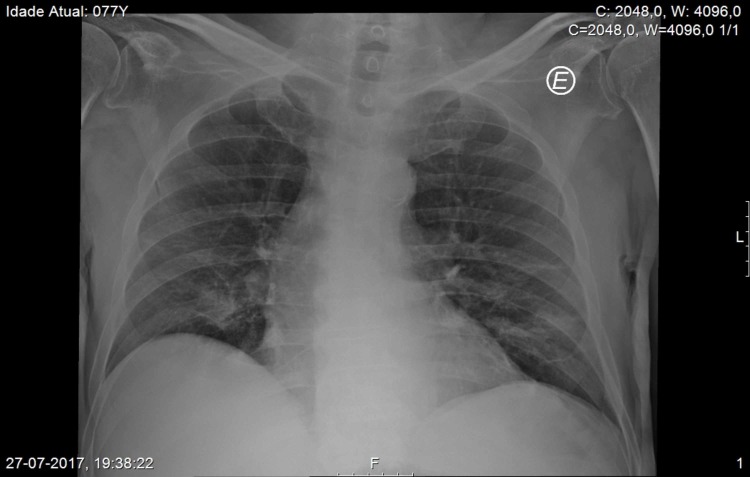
Chest X-ray, posteroanterior view Chest X-ray, posteroanterior view, on the second admission, showing residual image of organized cryptogenic pneumonia of the right lung, the patient had the year before
